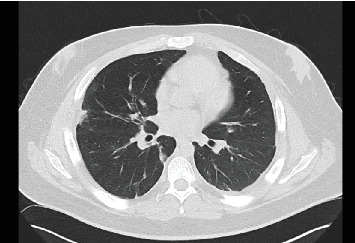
Transverse computed tomography thorax 3 months after presentation demonstrating residual small left pleural effusion, improvement in size of cavitations and resolving consolidation.
